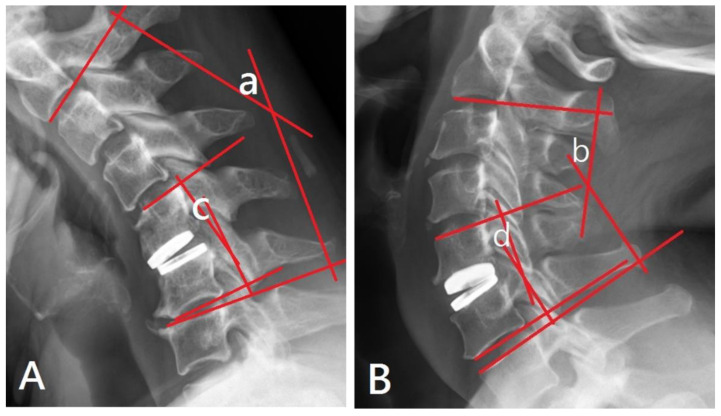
(A) (flexion view) and 1 (B) (extension view): Evaluation of the ROM of C2–C7 and FSU using flexion and extension lateral cervical radiographs. The angle of “a+b” represents the ROM of C2–C7. The angle of “c+d” represents the ROM of FSU.
